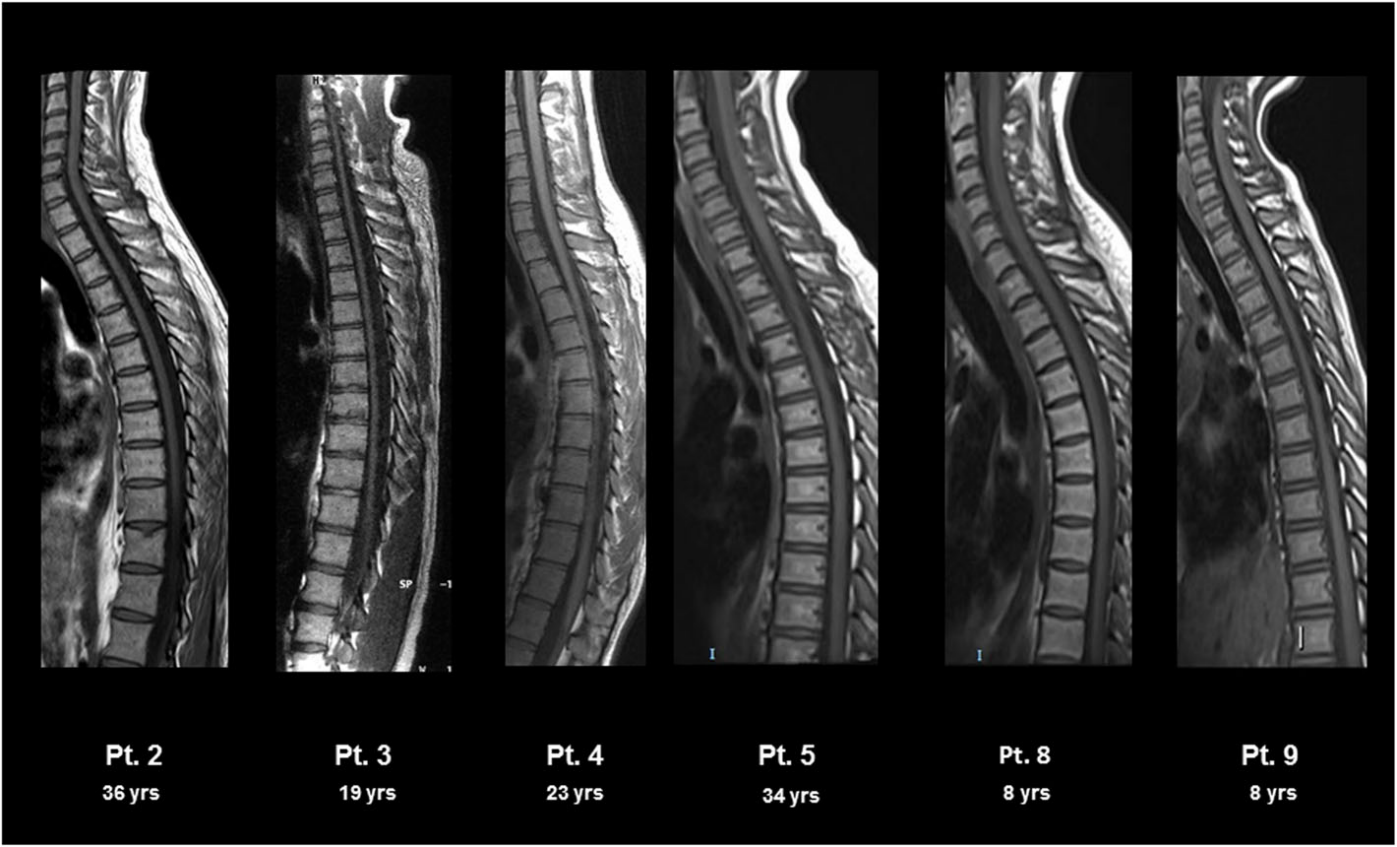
Spinal cord MRI in patients with HHH syndrome. T1 weighted spinal cord MRI showed atrophy of variable degree (severe in patients #3, moderate in patient #2, mild in patient #4, and absent in patients #5, 8 and 9)
